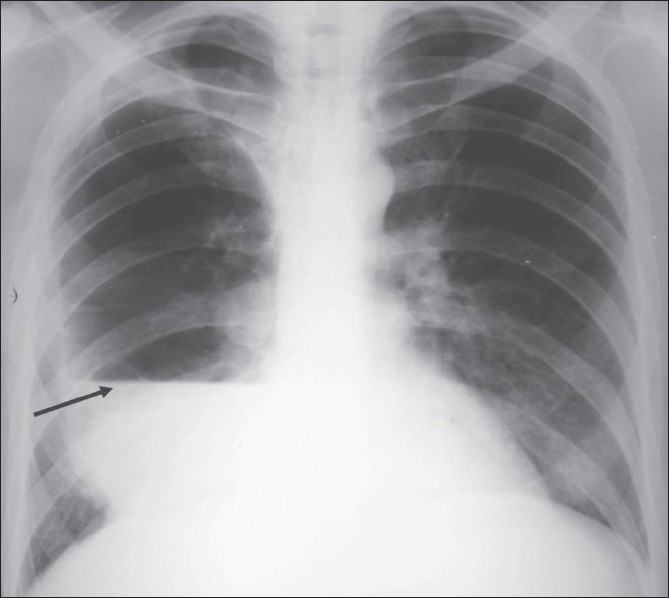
A chest radiograph at presentation shows a large air-fluid level in the right mid and lower zones (arrow), suggestive of an infected bulla
